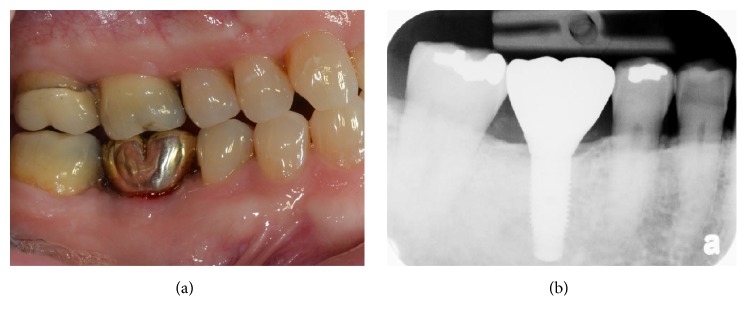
One-year follow-up after crown delivery. There is no evidence of inflammation or symptoms (a). Crestal bone loss or typical retrograde peri-implantitis was not noted on the periapical radiograph (b).
